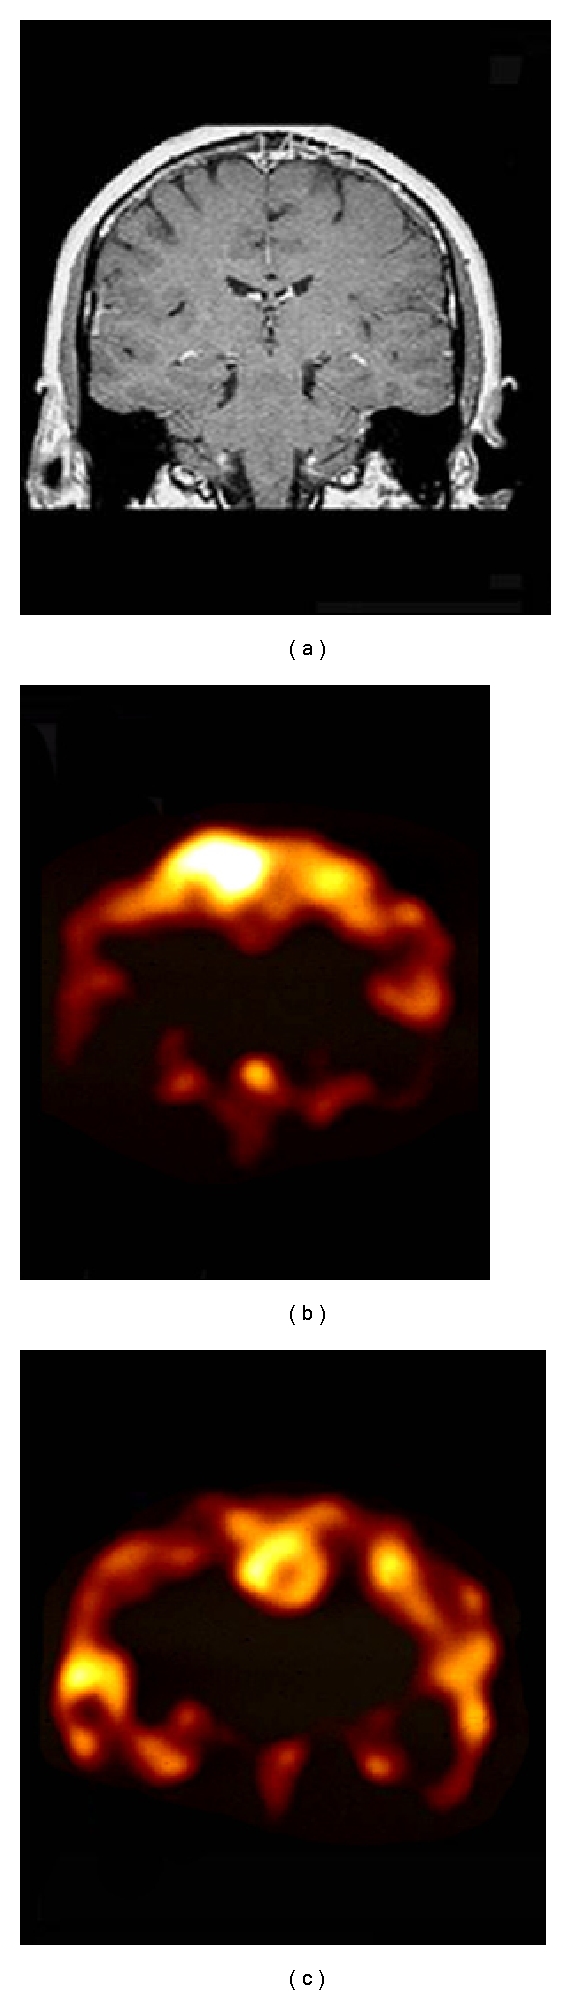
42-year-old female with intractable seizures with seizure activity frequency of 1-2 brief seizures per day, felt to arise from the frontal lobe regions, but were nonlocalizing by video-EEG monitoring. (a) The MRI scan is normal. (b) The ictal Tc-99m HMPAO brain SPECT scan showed a focal area of significant hyperemia in the right mesial frontal lobe. (c) There was minimal reduction of F-18 FDG uptake in this location, but this was not specific for the identification of the epileptogenic focus.
